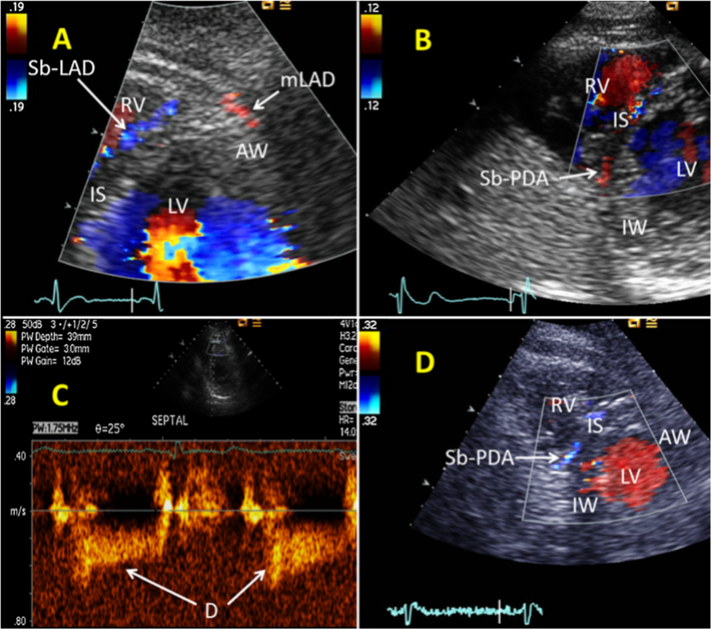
Examples of normal anterograde and abnormal retrograde blood flow in septal perforating branches. (A) In a modified parasternal short-axis view, a septal perforating branch from the left anterior descending coronary artery (Sb-LAD) is seen with anterograde blood flow. (B) In a modified parasternal short-axis view, a septal perforating branch from the posterior descending coronary artery (Sb-PDA) is seen with anterograde blood flow. (C) Spectral Doppler tracings of the anterograde blood flood in a Sb-LAD. (D) In a modified parasternal short-axis view, a Sb-PDA is seen with retrograde blood flow. AW = anterior wall of the left ventricle, D = spectral Doppler tracings of diastolic coronary blood flow, IS = interventricular septum, IW = inferior wall of the left ventricle, LV = left ventricle, mLAD = parts of the middle segment of the left anterior descending coronary artery, RV = right ventricle.
